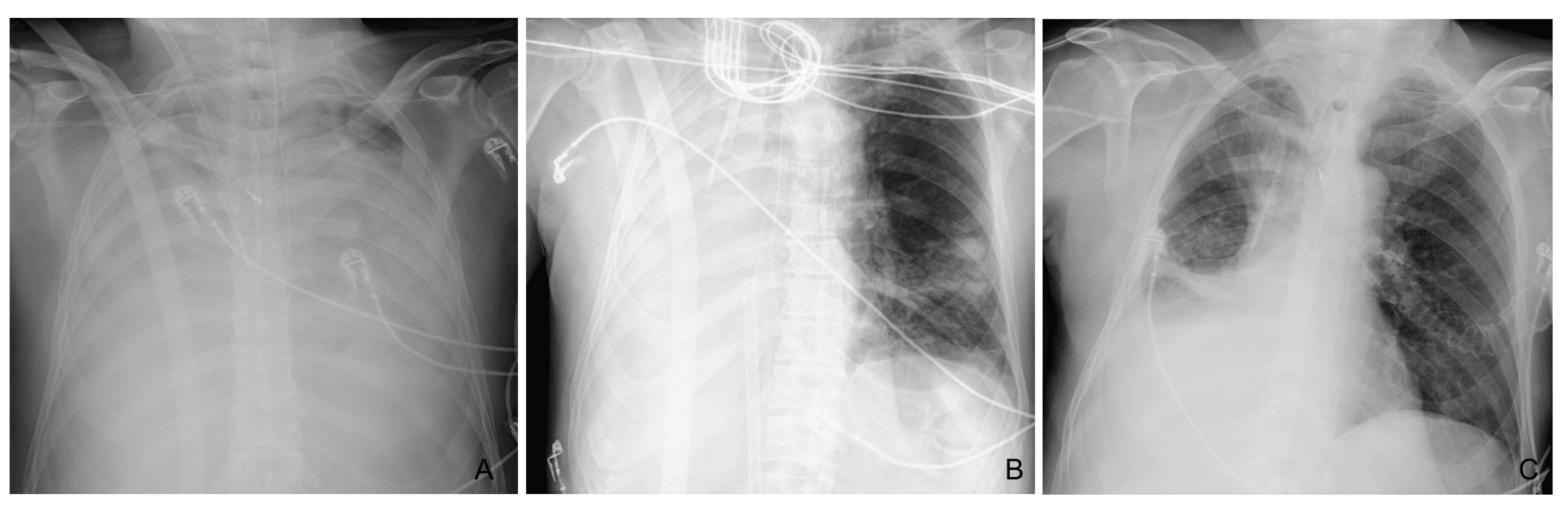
Chest radiograph showing (A) total opacification of both lung fields, (B) two days after right lower lobectomy, and (C) at the time of transfer
